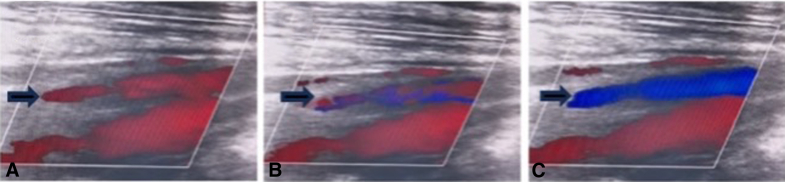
Color Doppler ultrasonographic imaging of the SFA. The SFA is indicated by the *arrow*, with the femoral vein situated inferiorly. Ultrasonography revealed bidirectional flow within the SFA: (A) The flow direction in the SFA was consistent with that in the femoral vein, indicating retrograde arterial flow; (B) transitional moment of bidirectional flow within the SFA; (C) distinct flow directions in the SFA and the femoral vein. *SFA*, Superficial femoral artery.
